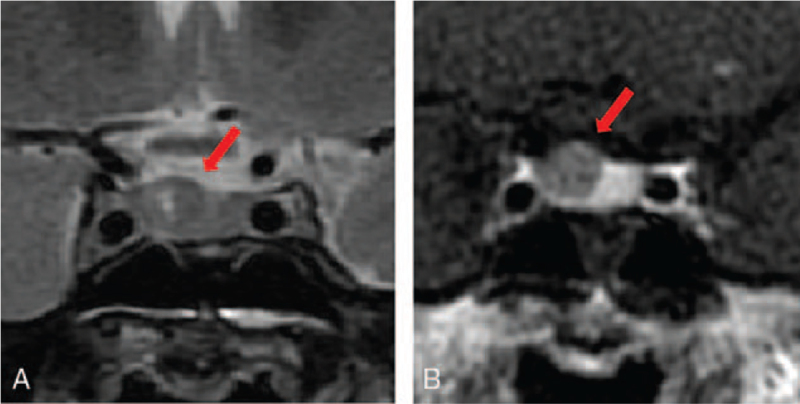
(A) In brain contrast-enhanced magnetic resonance imaging (MRI), nodular lesion was observed in the pituitary gland and its diameter was approximately 10 mm. (B) In dynamic study, the nodule lesion was slightly contrasted, but contrast effect was weaker compared to the adjacent pituitary gland.
